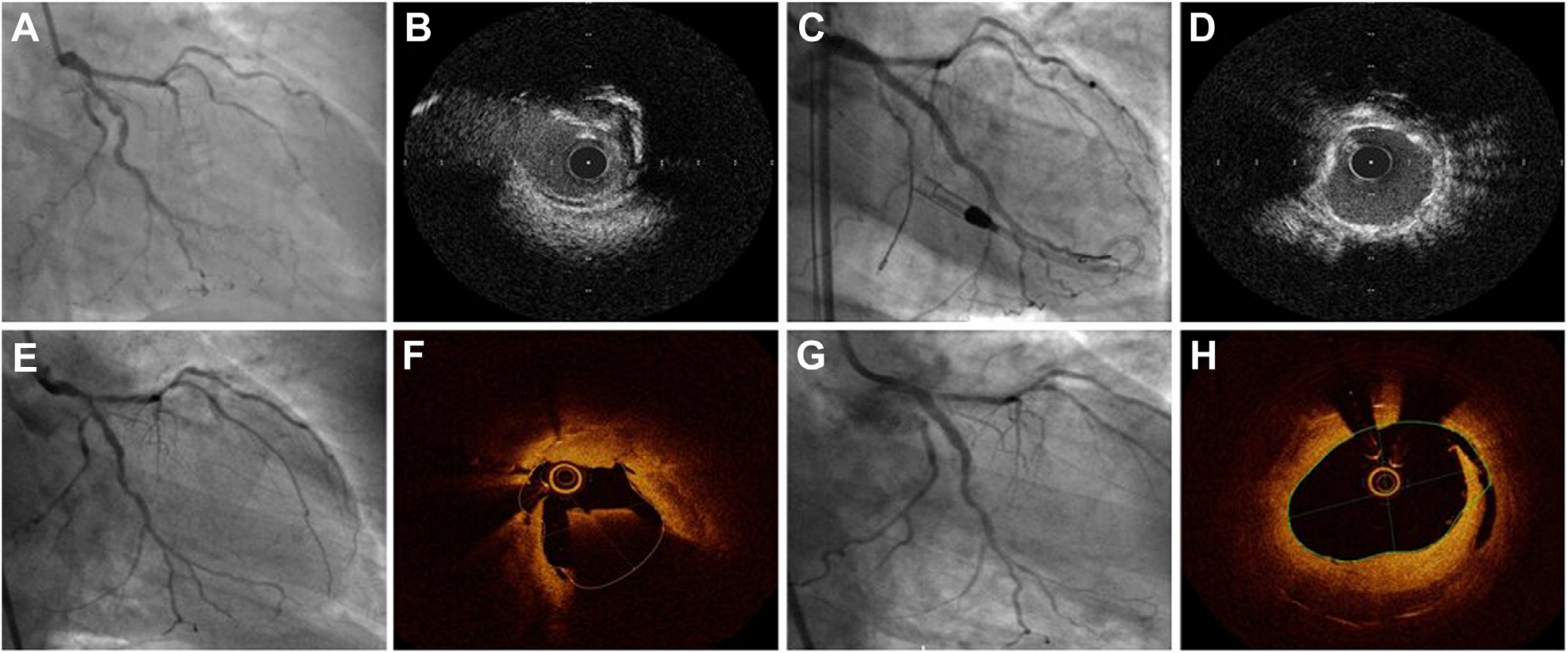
Coronary Angiography and Intravascular Imaging (A) Baseline coronary angiography showing severe calcific disease in the distal left main stem and proximal left circumflex. (B) Intravascular ultrasound image demonstrating protruding calcific nodule at the ostium of the left circumflex. (C) Postintervention coronary angiography. (D) Postintervention intravascular ultrasound of well-expanded stent at the site of the nodule. (E) Coronary angiography following presentation with recurrent acute coronary syndrome. (F) Optical coherence topography following predilation demonstrates calcific nodule protruding through the stent in the distal left main stem. (G) Coronary angiogram following rotational atherectomy and treatment with drug-coated balloon. (H) Postintervention OCT showing successful ablation of the nodule and dissection within the neointimal tissue.
